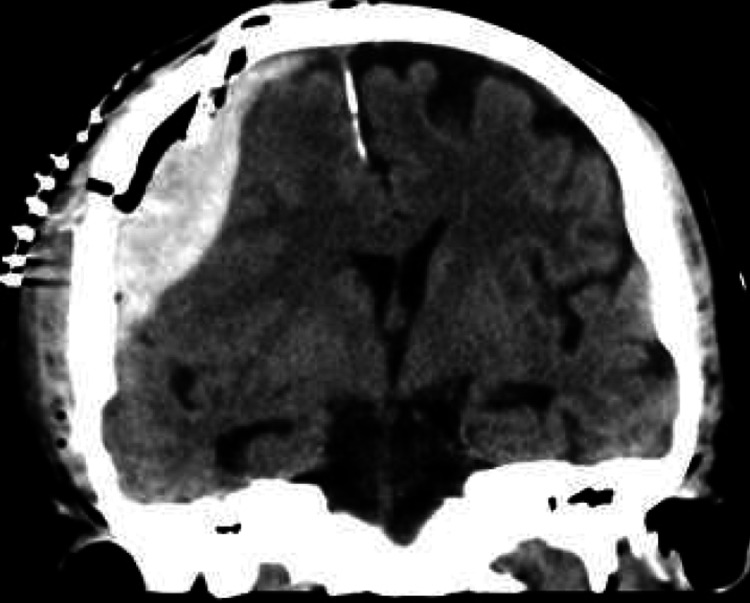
Coronal CT scan showing hyperdense subdural hematoma, partially mixed with air on the right side.
